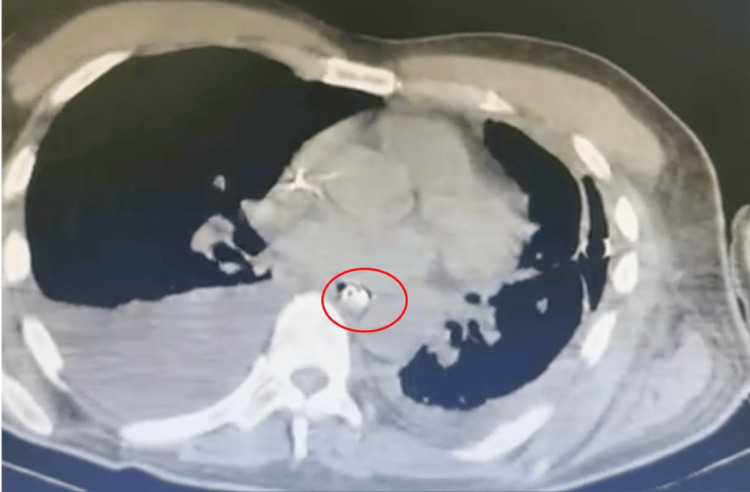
Bilateral pleural effusion and esophageal vacuum therapy catheter on CT. Chest computed tomography showing bilateral pleural effusion and an intraluminal esophageal catheter for vacuum therapy (red circle).
